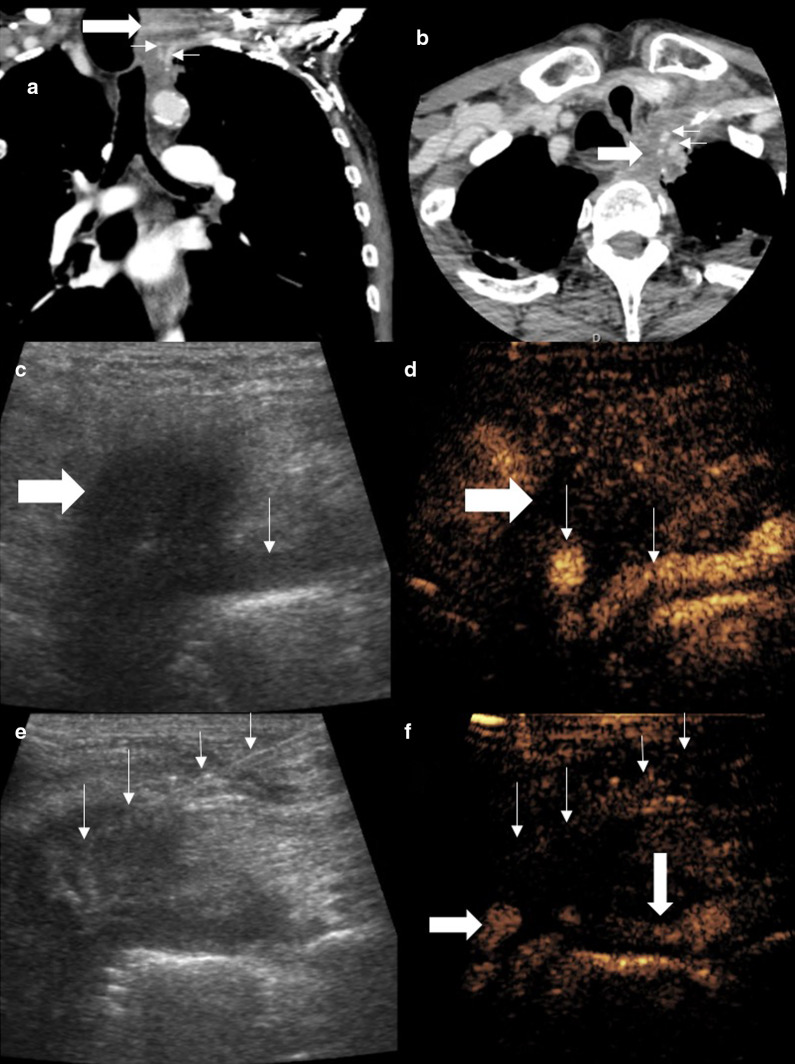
Patient with Pancoast adenocarcinoma of the lung. Coronal (a) and axial (b) views show soft tissue density (thick arrows) encasing the left carotid artery and subclavian artery (thin arrows). c The tumour is seen as a hypoechoic area, ill-defined on B mode ultrasound with the subclavian artery visible on the periphery (thin arrow). d Simultaneous CEUS clearly defines the hypoenhancing lesion and the previously unseen carotid artery and subclavian artery (thin arrows). e, f Simultaneous B mode ultrasound and CEUS with the biopsy needle (thin arrows) within the hypoenhancing lesion avoiding the critical arterial structures (arrow)
